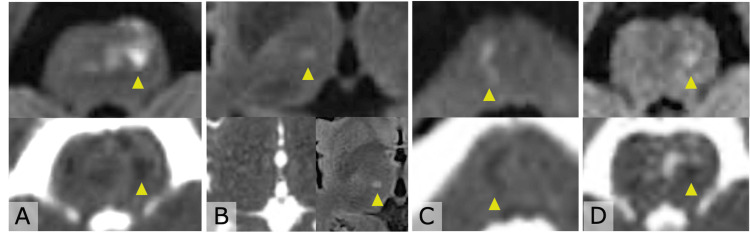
Serial diffusion-weighted MRI imaging (DWI) and apparent diffusion coefficient (ADC) maps of four recurrent cerebral infarctions. Panel A shows the first, panel B the second, panel C the third, and panel D the fourth cerebral infarction. (A), (C), and (D): (top) DWI shows restriction in the left pons (A, D) or right pons (C); (bottom) the ADC map shows low signal. (B): (top) DWI shows restriction in the right thalamus; (bottom left) the ADC map is isointense; (bottom right) fluid-attenuated inversion recovery shows hyperintensity, consistent with MRI findings of an infarction approximately one week after onset. Yellow arrowheads indicate the location of each infarct.
